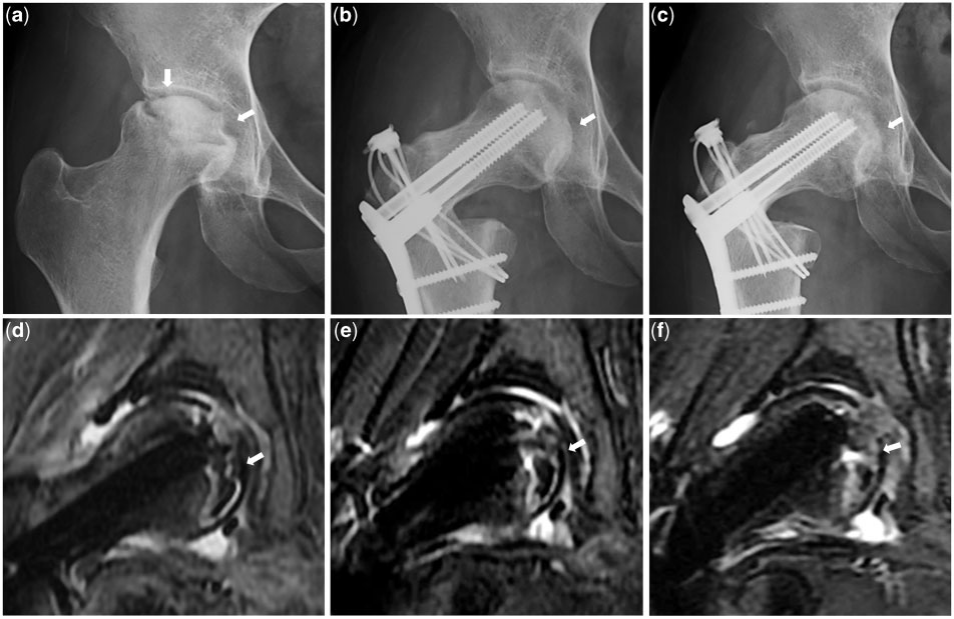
(a) Preoperative plain AP X-ray. (b) AP X-ray 1 month after operation. (c) One year after operation. (d) MRI T2 fat suppression coronary image 1 month after operation. (e) 6 months after operation. (f) One year after operation.
